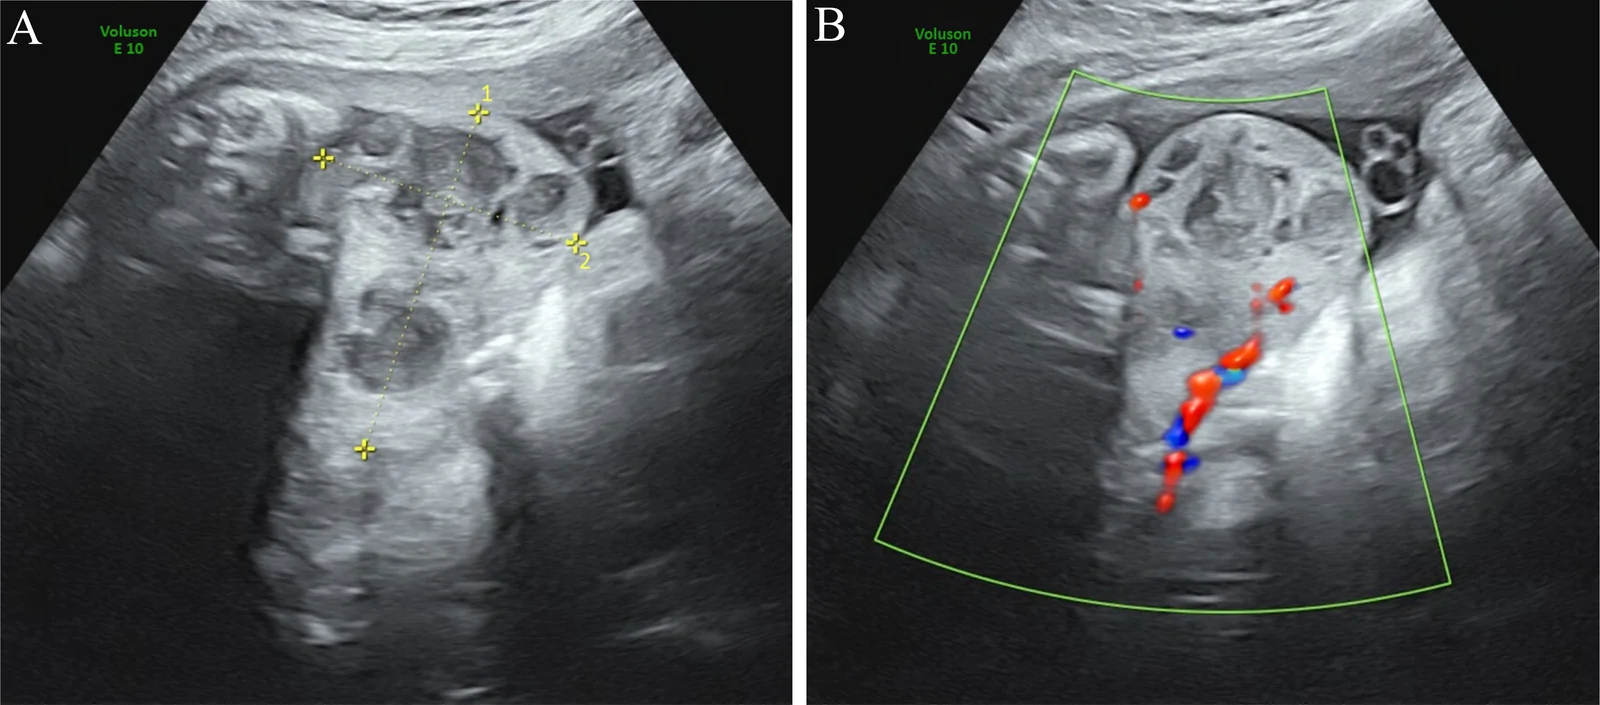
Prenatal US at 38 weeks. (A) Image shows a 5.0×4.1cm solid mass in the oral cavity protruding outward. (B) Color Doppler image demonstrates abundant internal blood flow.
